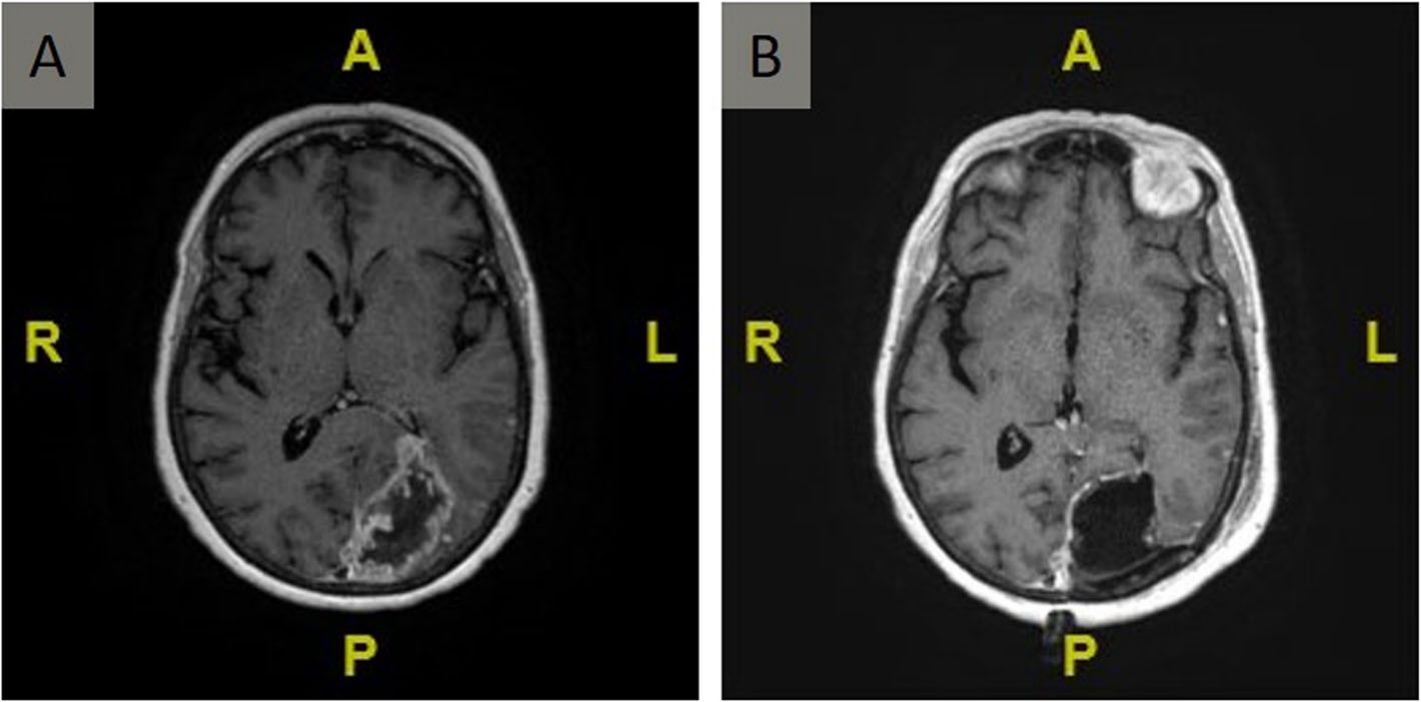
Primary GBM in the left occipital lobe. Axial T1 gadolinium-enhanced magnetic resonance image performed (a) preoperatively, and (b) 24 h postoperatively
